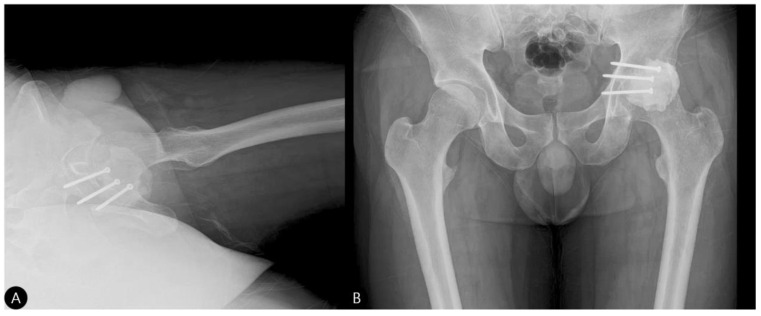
Three-month postoperative follow-up radiographs. (A) Cross-table lateral view showing a stable hip joint without subluxation. (B) AP view of the hip demonstrating a maintained joint space without evidence of screw migration.
